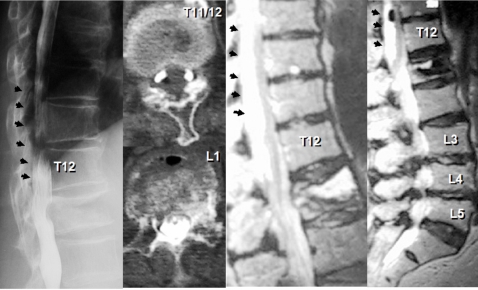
A case of a 78-year-old female. Preoperative radiographic examinations revealed L1 osteoporotic vertebral collapse with an intravertebral cleft, dorsal epidural soft tissue mass causing posterior spinal cord compression at T9-T12 levels (arrow), and severe degenerative canal stenosis at L3-L4 and L4-L5. Both intravertebral cleft and epidural soft tissue mass contained some amount of gas inside.
